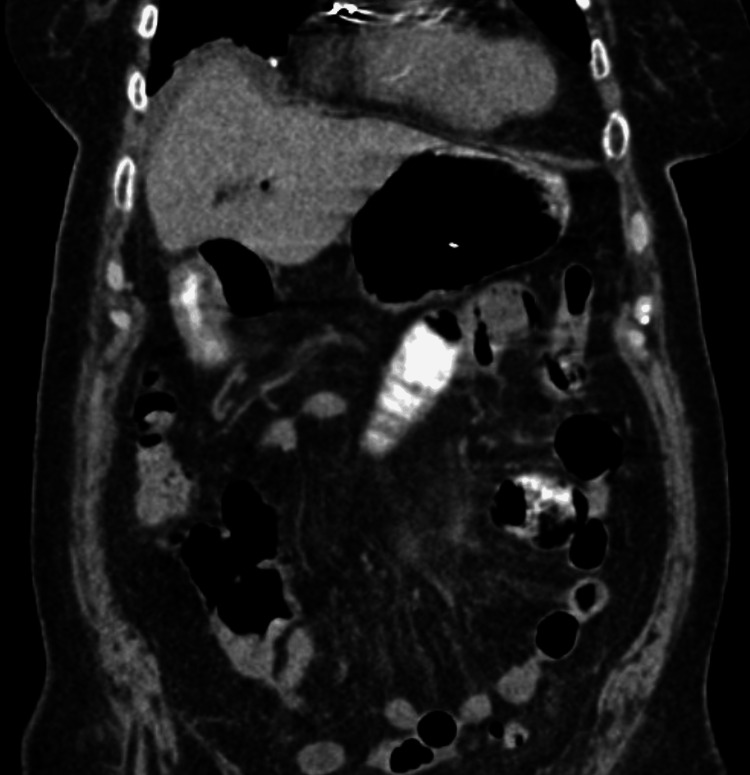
Computed tomography showing pneumobilia.
